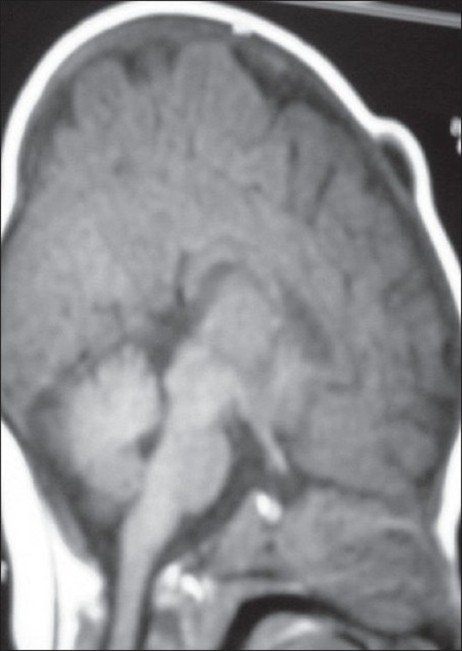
T1-Weighted image, sagittal view of brain MRI of a two month-old infant reveals hypointense mass around the anterior fontanel with intact bone under the lesion
